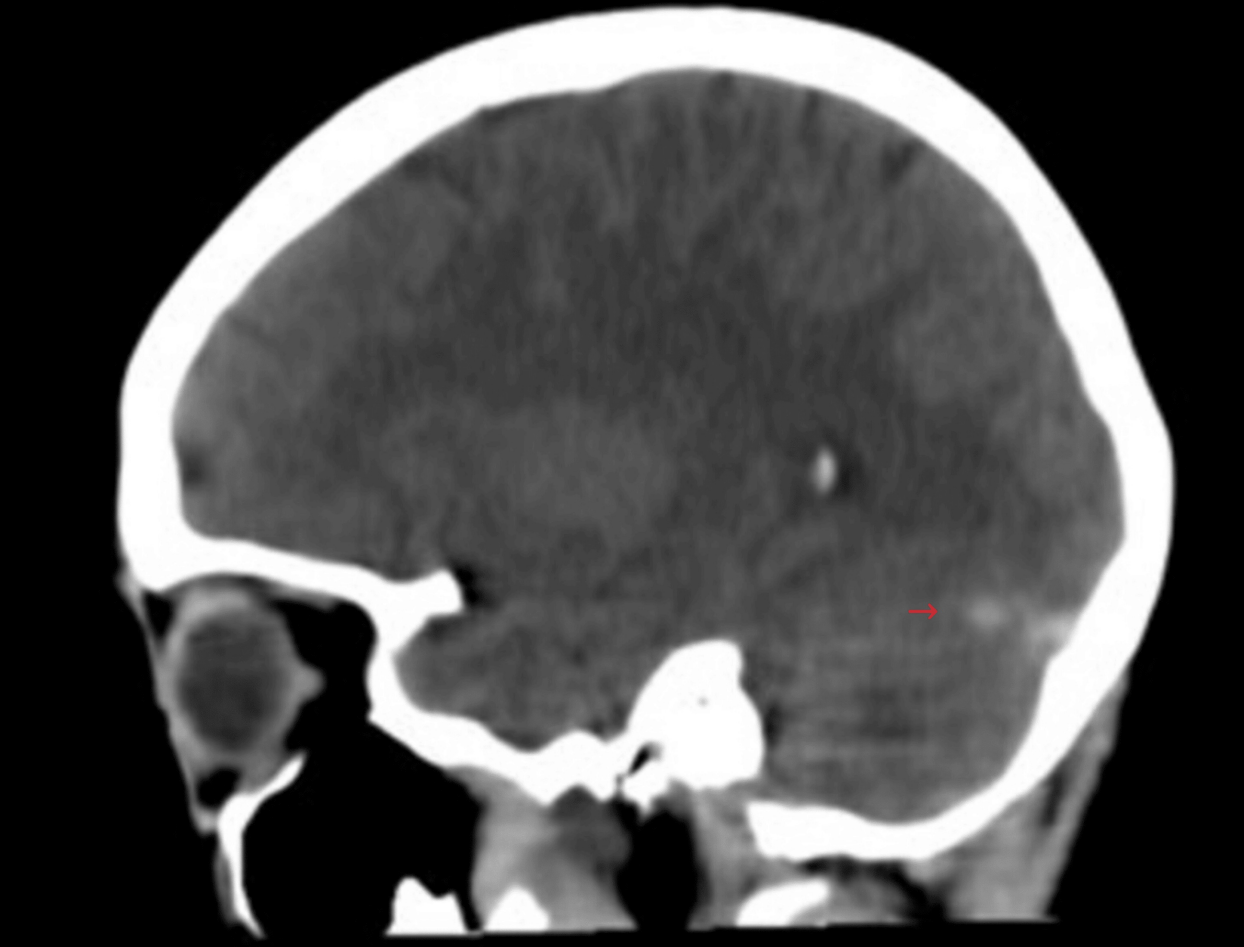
Sagittal CT slicing of the single skull Hyperdensity of the right transverse sinus
